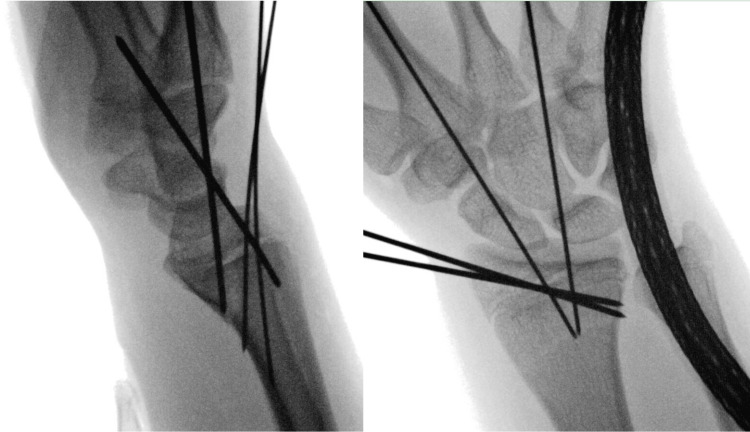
Operative fracture reduction and fixation Radiographs showing acceptable alignment after closed reduction and percutaneous pinning of the fracture.
